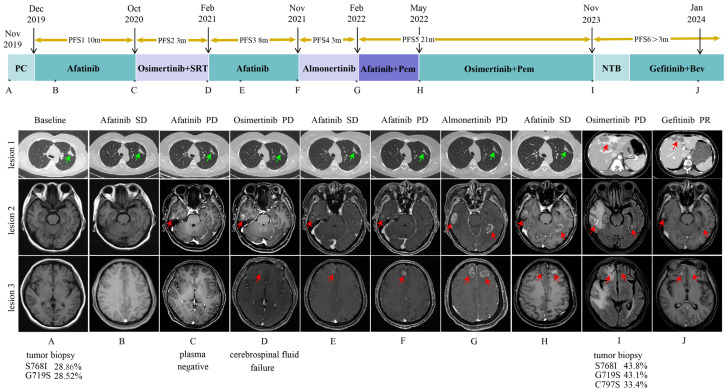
A summary of the patient’s clinical course. The upper panel shows the various treatments the patient received for *EGFR*-mutated non-small-cell lung cancer as well as the duration of each treatment. Asterisks indicate the time points for the response assessments. Green arrows indicate the primary lesion, while red arrows indicate the metastatic lesions. Genetic testing results for *EGFR* of the various tissue biopsies are shown below the corresponding CT images with their minor allele frequency. (A) Baseline chest CT scan and head MRI at diagnosis in November 2019. (B) SD on afitinib treatment in February 2020. (C) Lung lesion SD but head lesions PR on afitinib treatment in October 2020. (D) Lung lesion SD but head lesions PR on osimertinib treatment in February 2021. (E) SD on afitinib treatment in April 2021. (F) Lung lesion SD but head lesions PR on afitinib treatment in November 2021. (G) Lung lesion SD but head lesions PR on almonertinib in February 2022. (H) SD on afitinib treatment in May 2022. (I) Liver lesion and head lesion PD on osimertinib combined with pemetrexed treatment in November 2023. (J) PR on gefitinib and bevacizumab treatment in January 2024. Abbreviations: PFS, progression-free survival; PC, pemetrexed + cisplatin; SRT, stereotactic radiotherapy; Pem, pemetrexed; NTB, nab-paclitaxel + tirellizumab + bevacizumab; Bev, bevacizumab; SD, stable disease; PD, progressive disease; PR, partial response.
